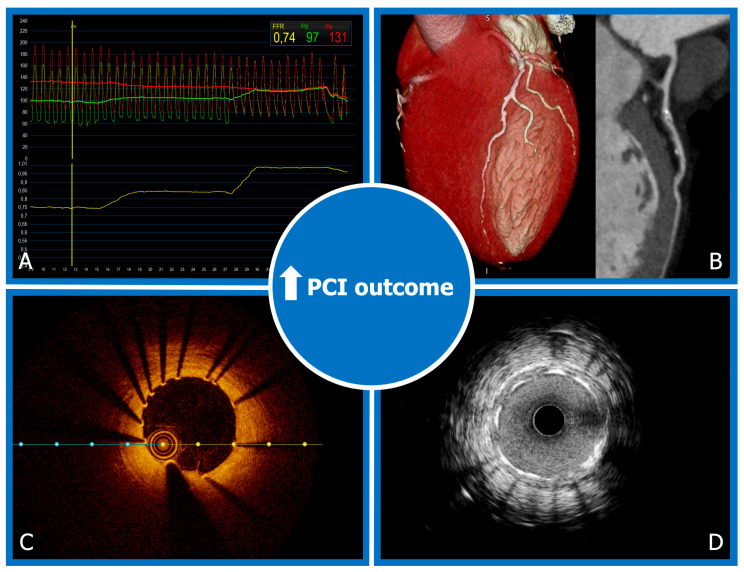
PCI optimization strategies to improve clinical outcome. Physiology assessment with FFR pullback (A). Coronary CTA showing anatomical 3D and multiplanar reconstructions (B). Post-PCI OCT and IVUS evaluation of stent expansion and apposition (C,D).
